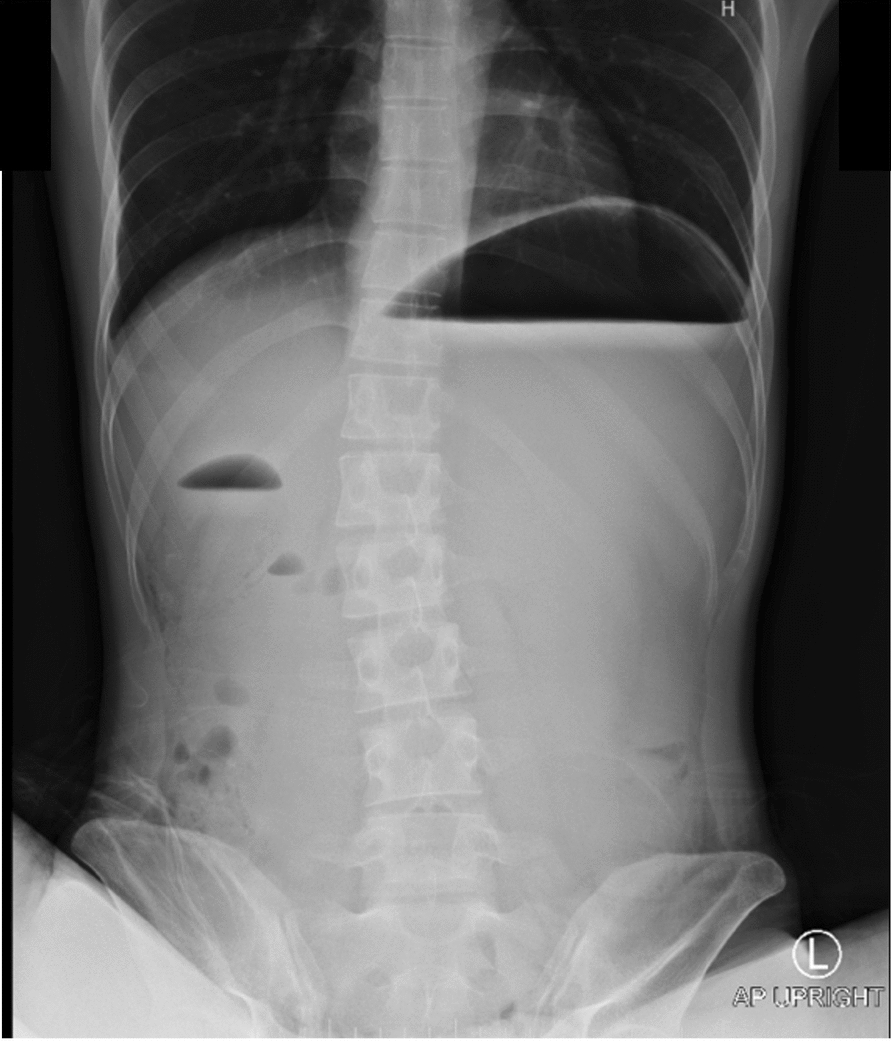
Plain upright abdominal radiograph showing dilated stomach and duodenum
